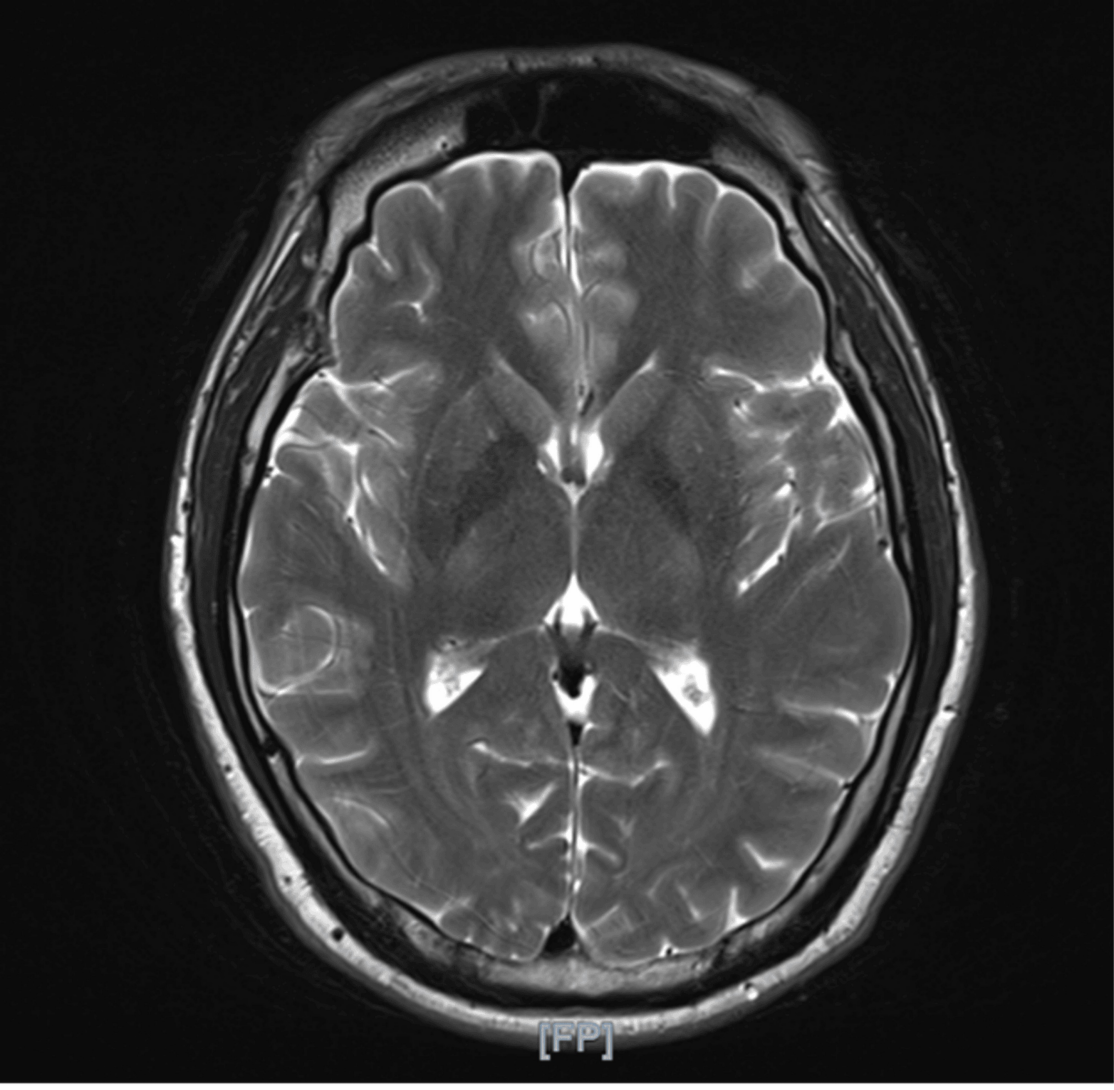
Axial T2-weighted MRI of the brain demonstrating no acute pathology. No evidence of stroke, demyelination, or basal ganglia lesions.
